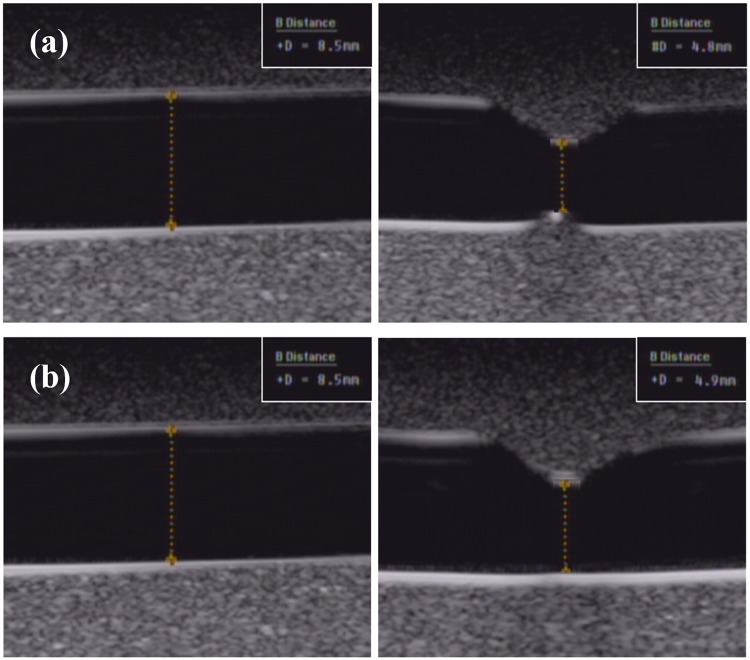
Ultrasound B-mode images of contraction in vessel mimic substance; (a) non-BMF flow, (b) BMF flow.
